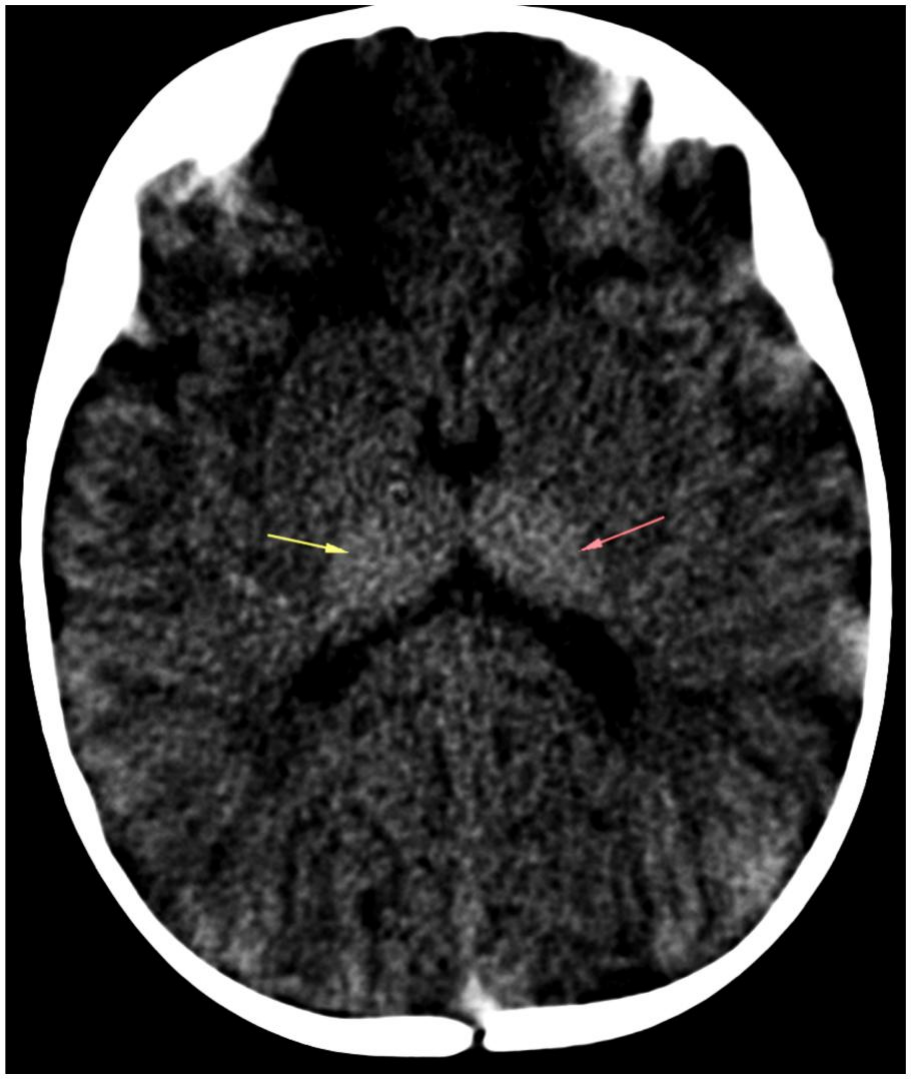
Axial NCCT image demonstrating bilateral hyperdense thalami (arrows). Abbreviation: NCCT = non-contrast CT.
